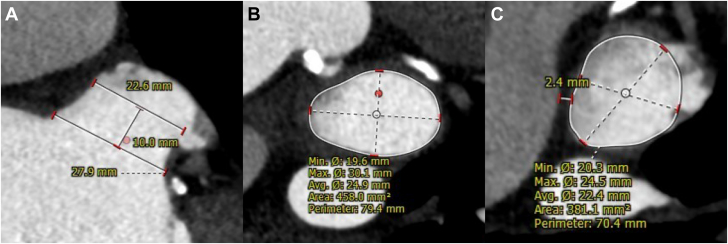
Cardiac CT Imaging for LAA Screening Cardiac CT revealed (A) a small, chicken wing–shaped LAA with sufficient depth for device deployment and no evidence of thrombus. The LAA measured (B) 30.1 × 19.6 mm at the ostium and (C) 24.5 × 20.3 mm at the planned landing zone. (C) At the landing zone, the distance between the pulmonary artery and the LAA was 2.4 mm. CT = computed tomography; LAA = left atrial appendage.
